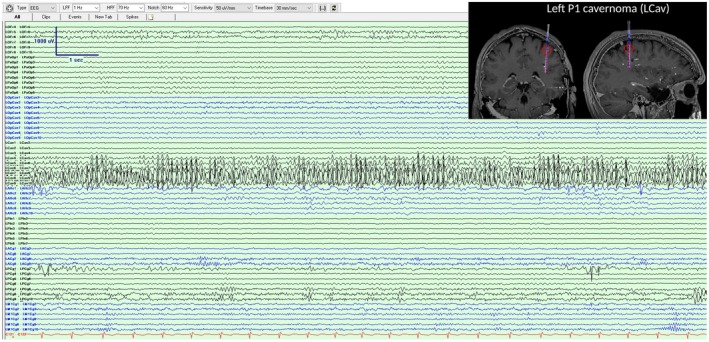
SEEG recording demonstrating abundant sharply contoured 13 Hz activity over LCav 7–10. Inset of coronal and sagittal MRI brain showing the left parietal cavernoma and the position of the SEEG electrode close to it, with affected contacts circled in red.
